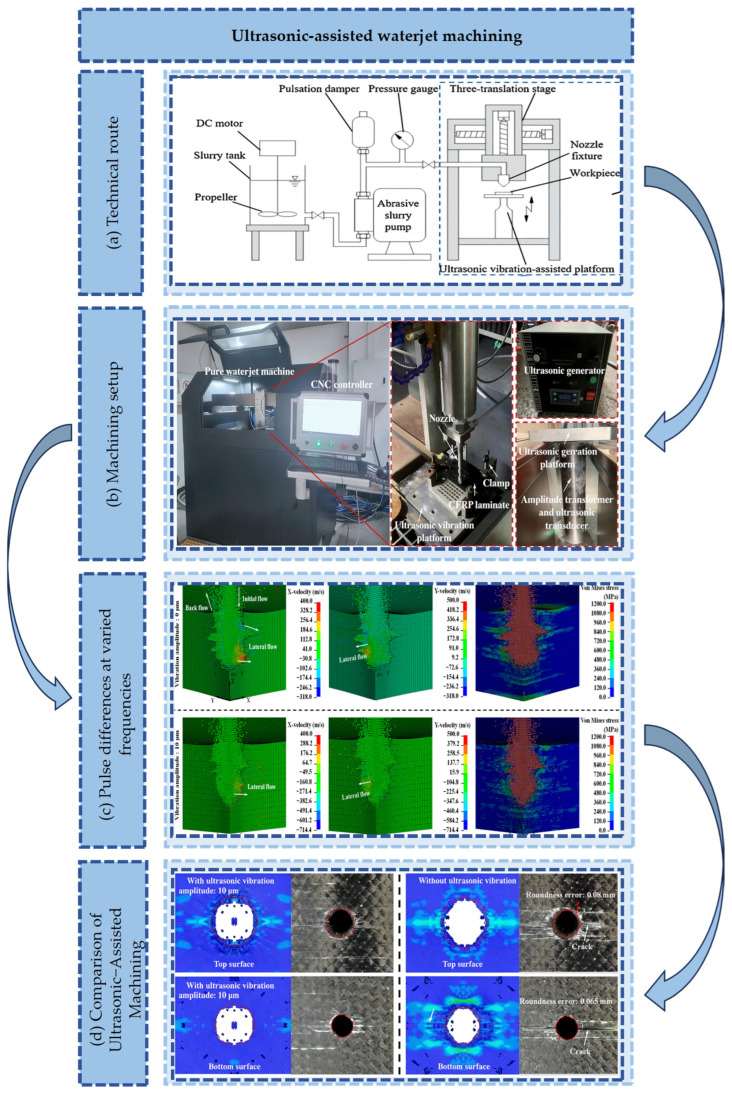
Ultrasonic−assisted waterjet machining: (a) Technical route [22]. (b) Machining setup [76]. (c) Pulse differences at varied frequencies [76]. (d) Comparison of Ultrasonic-Assisted Machining [76].
